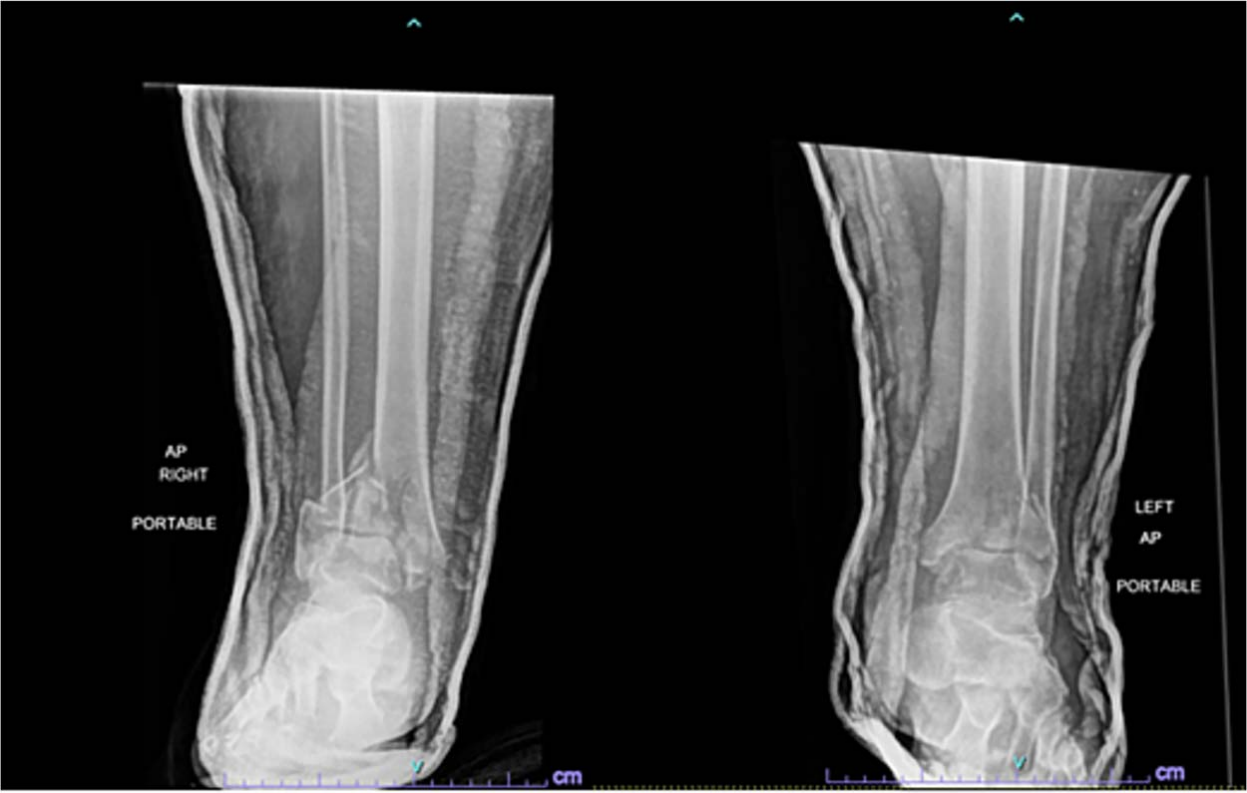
Anterior-to-posterior (AP) radiographs of patient 1 obtained 10 days after injury, upon transfer to our facility, demonstrating bilateral pilon fractures.
